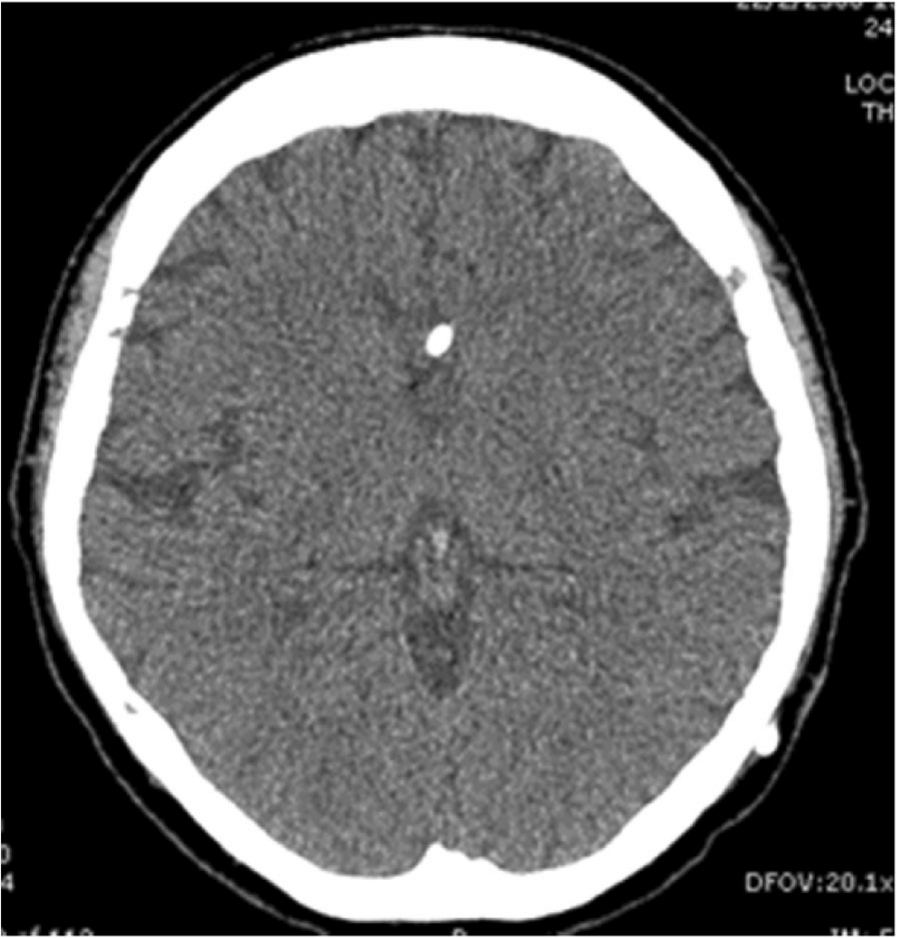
Computed tomography of brain following the shunt reimplantation exhibited significantly decreased ventricular dilatation
